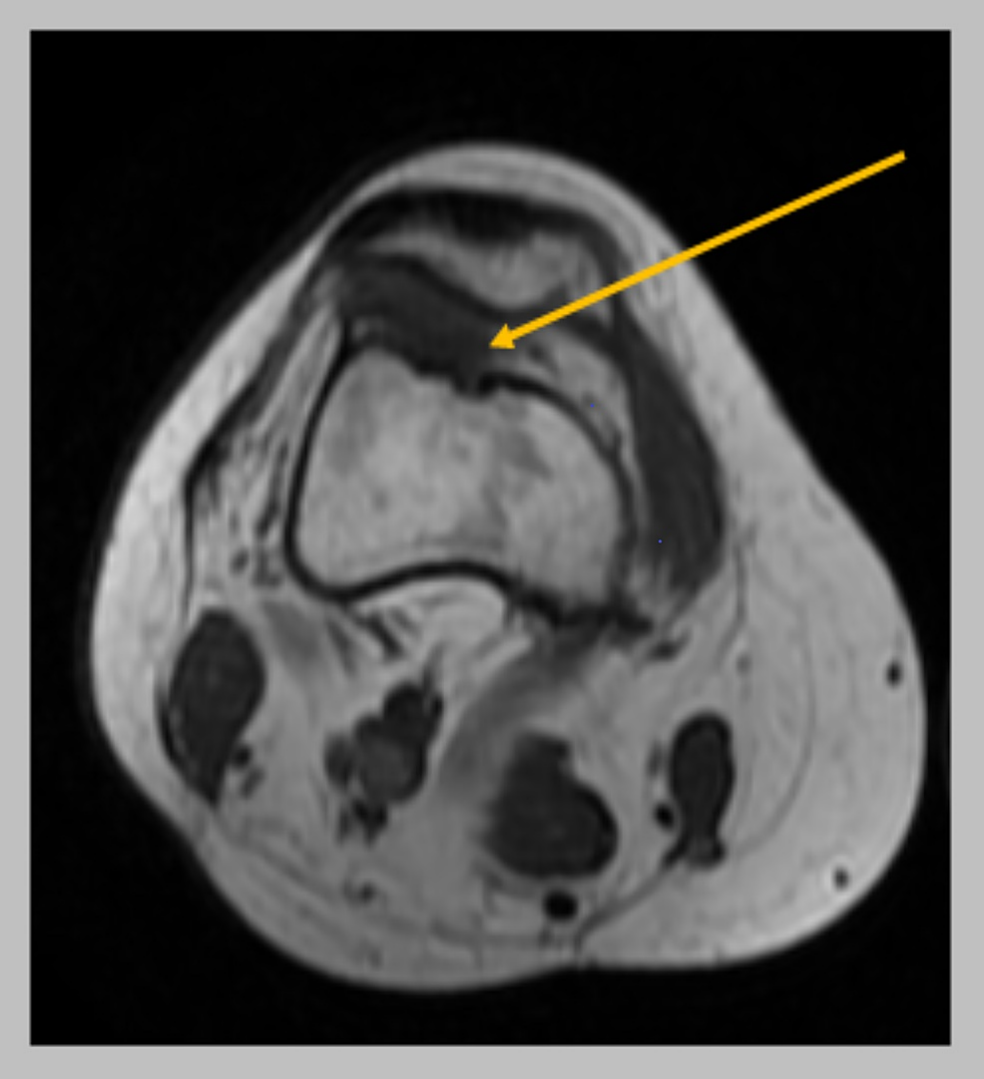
MRI transverse plane showing the lesion (yellow arrow).
